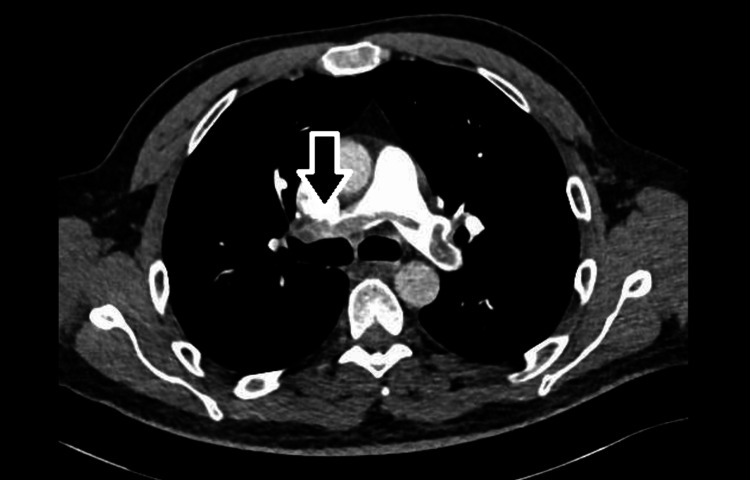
CT pulmonary angiography CT pulmonary angiography protocol, at the bifurcation of pulmonary trunk showing the filling defect (arrow).
